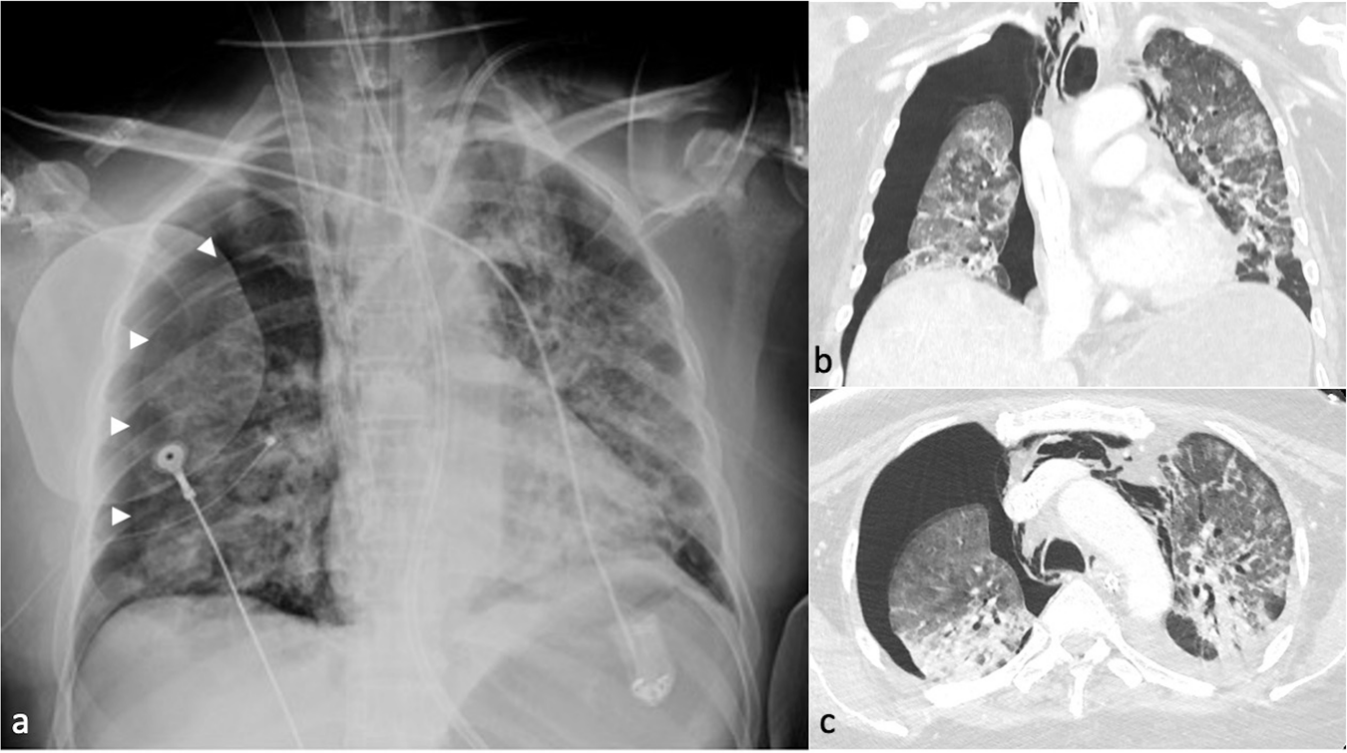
Femoro-atrial veno-venous (VV) extracorporeal membrane oxygenation (ECMO) in a patient with bilateral interstitial pneumonia due to SARS-CoV-2 infection. Chest X-ray (CXR) (a) after cannulation shows a significant right pneumothorax with partial lung collapse (arrowhead) and pneumomediastinum, particularly in the upper and mid-third of the mediastinum on the right, near the para-caval area. The distance between the cannula tips is less than 8 cm. Chest computed tomography (CT) in lung window setting (b, c) confirms the right pneumothorax, causing partial collapse of the right lung, and pneumomediastinum
